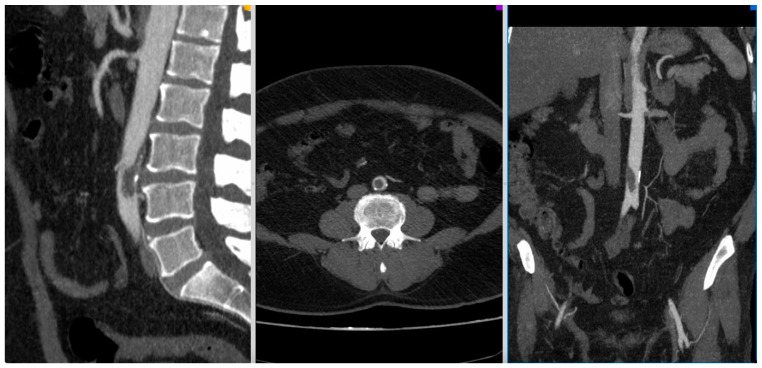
CT-Angio of a 52 yo patient with aortic thrombosis with acute right lower limb ischemia 8 days after SARS-CoV-2 diagnosis.
